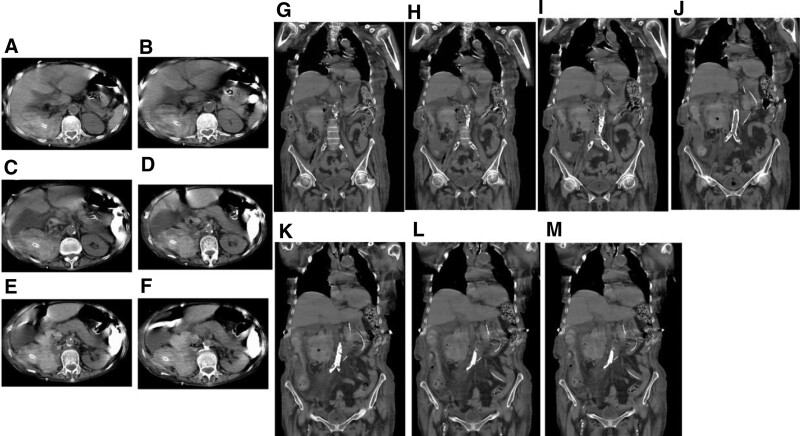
Abdominal computed tomography (CT) images after radical right nephrectomy/pyonephrosis-nephrectomy and ureteral stump resection in the patient. Abdominal CT imaging was performed following radical right nephrectomy and ureteral stump resection, and it revealed multiple instances of pneumoperitoneum and high-density shadows, suggesting a hematoma and gastrointestinal (GI) bleeding in the patient.
